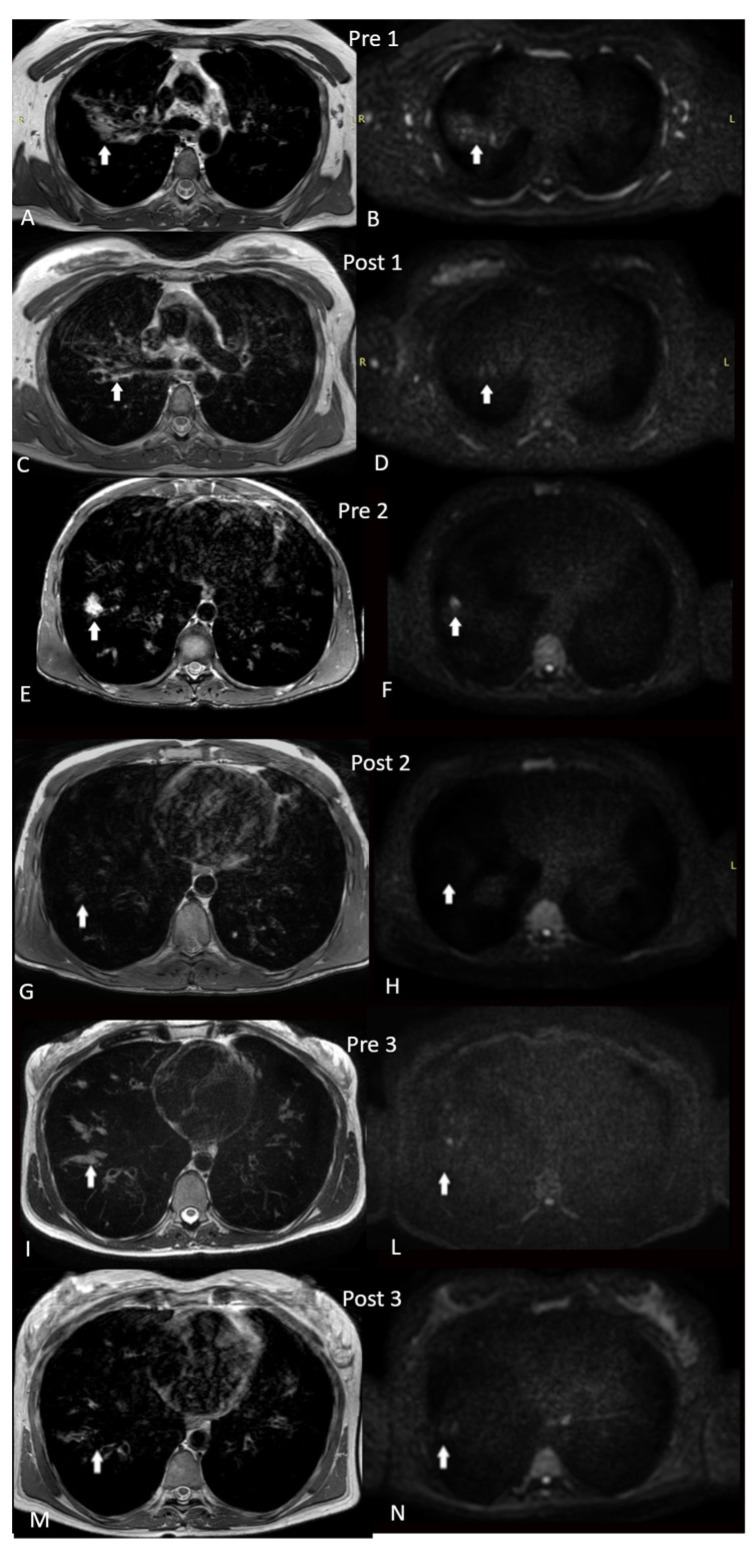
Patient 1, MRI images pre ELX/TEZ/IVA therapy: axial PD weighted image showing cilindric bronchiectasis with large amount of mucus plugging within and bronchial wall thickening (A). Atelectasis visible in the dorsal segment of the right upper lobe (A); DWIBS sequence at 800 b-value, with evidence of signal restriction in the mucus plugging (B). Patient 1, MRI images after 3 months of ELX/TEZ/IVA therapy: reduction in mucus plugging, and bronchial wall thickening (C); on DWIBS sequence at 800 b-value, no evidence of signal restriction (D). Patient 2, MRI images pre ELX/TEZ/IVA therapy: T2 axial image showing consolidation in the lateral segment of lower right lobe (E), with signal restriction in 800 b-value DWIBS (F). Patient 2, MRI images after 3 months of ELX/TEZ/IVA therapy: T2 axial image showing no consolidation (G) and no restriction in DWIBS sequence (H). Patient 3, MRI images pre ELX/TEZ/IVA therapy: PD weighted image, axial, showing evidence of mucus plugging on lateral segment of right lower lobe with bronchial wall thickening (I). Axial 800 b-value DWIBS sequence, showing signal restriction within the large mucus plugging (L). Patient 3, MRI images after 3 months of ELX/TEZ/IVA therapy: PD weighted axial, evidence of detersion of large airways mucus plugging of the bronchiectasis of the lateral segment of the lower right lobe, reduction in bronchial wall thickening (M). No evidence of signal restriction at 800 b-value DWIBS at the same level (N). Arrows were used to indicate the areas of interest.
